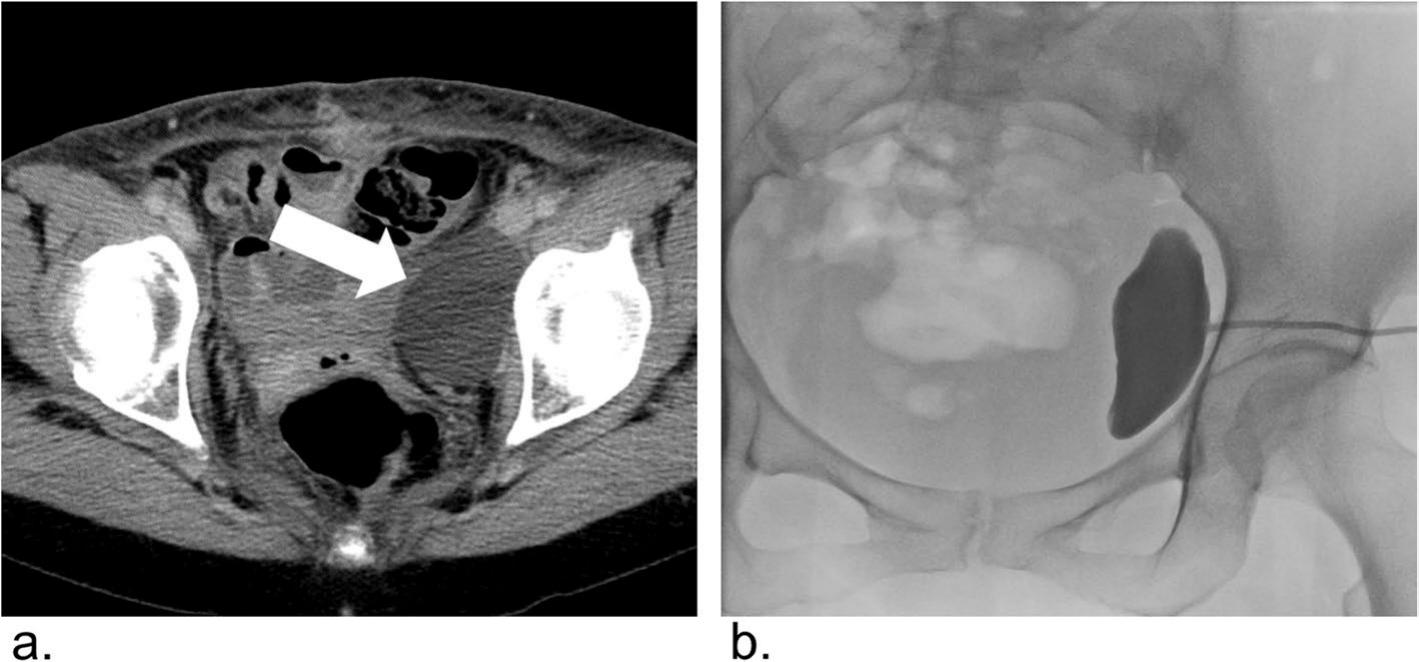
a Contrast-enhanced computed tomography (CT) after gynecological cancer surgery in a 60-year-old woman presenting with left low leg lymphedema. CT shows left pelvic lymphocele (white arrow). b Cavitogram shows no leakage of the lymphocele
